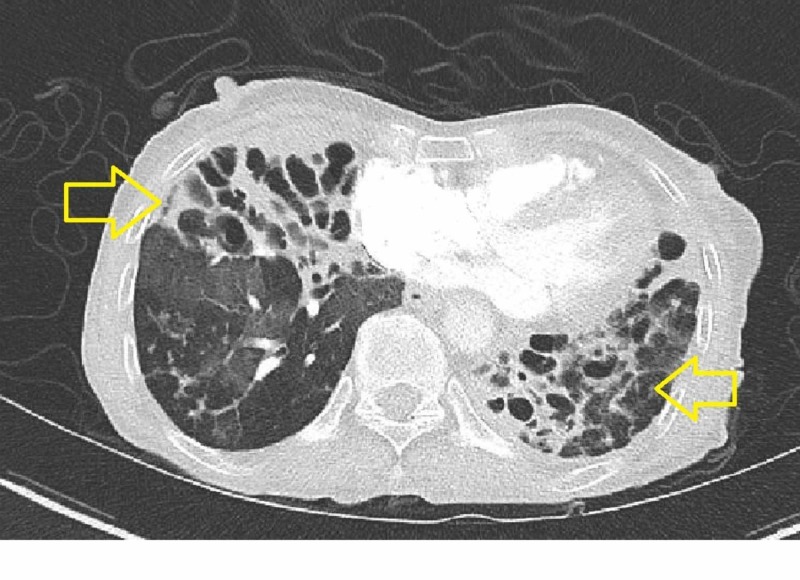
Computed tomography chest with IV contrast (axial) demonstrating bilateral cystic bronchiectasis with fibrosis indicative of chronic, advanced M. abscessus infection.
